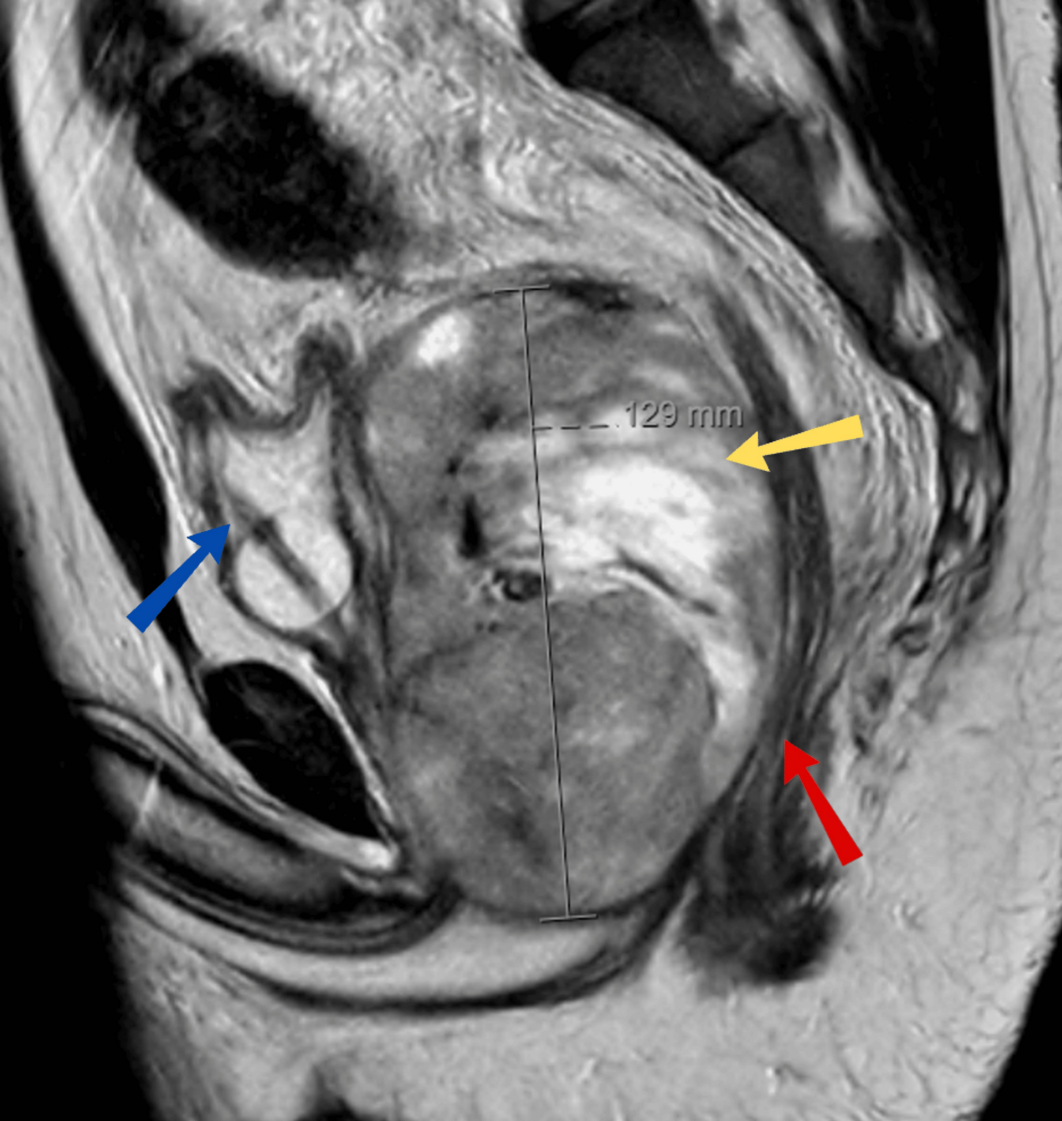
MRI pelvis with contrast demonstrating a large mass (yellow arrow) between the bladder (blue arrow) and rectum (red arrow)
